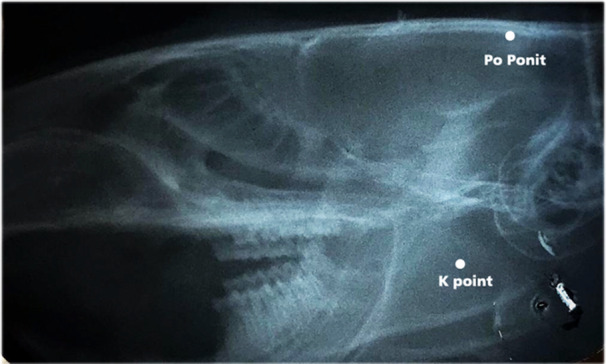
Selected points on the lateral skull radiographs used for optical bone densitometry.
